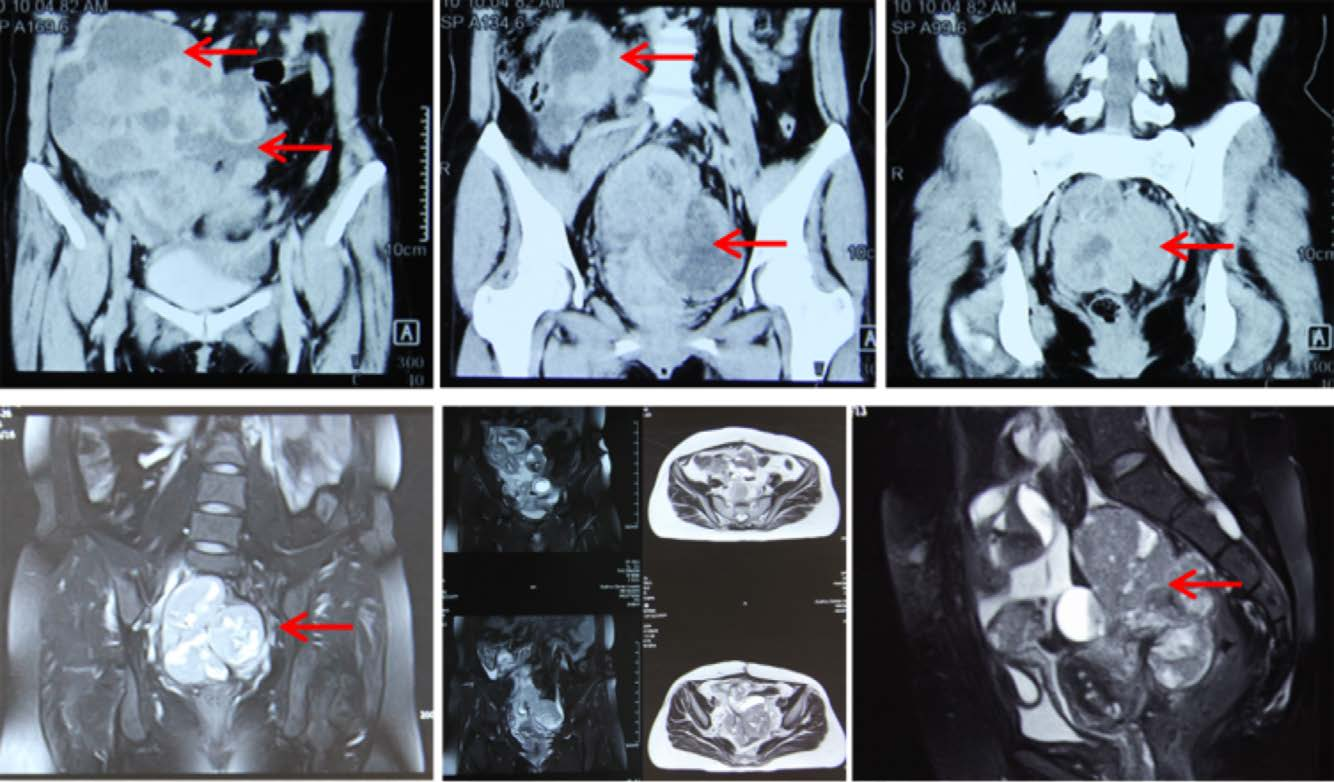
Abdominal magnetic resonance imaging showed a huge cystic-solid space from the right lower abdomen to the pelvis, with intratumoral hemorrhage, suspicious abdominal dissemination, and suspicious malignant lesions (December 2017).
